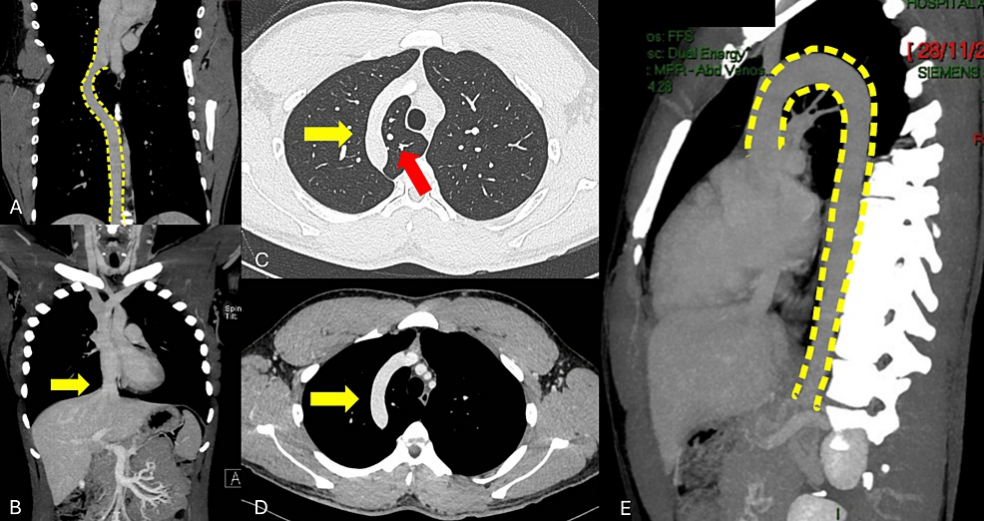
Contrasted thoracoabdominal computed tomography A-B: Anomalous dilation of the azygos vein (16.8 mm) (intermittent yellow line), associated with interruption of the inferior vena cava in the intrahepatic and adrenal portion (yellow arrow). C-D: Presence of dilation of the azygos vein (yellow arrow) in a transversal plane with the presence of an azygos pulmonary lobe (red arrow). E: Continuation through the azygos system (intermittent yellow line) and drainage of the superior vena cava.
